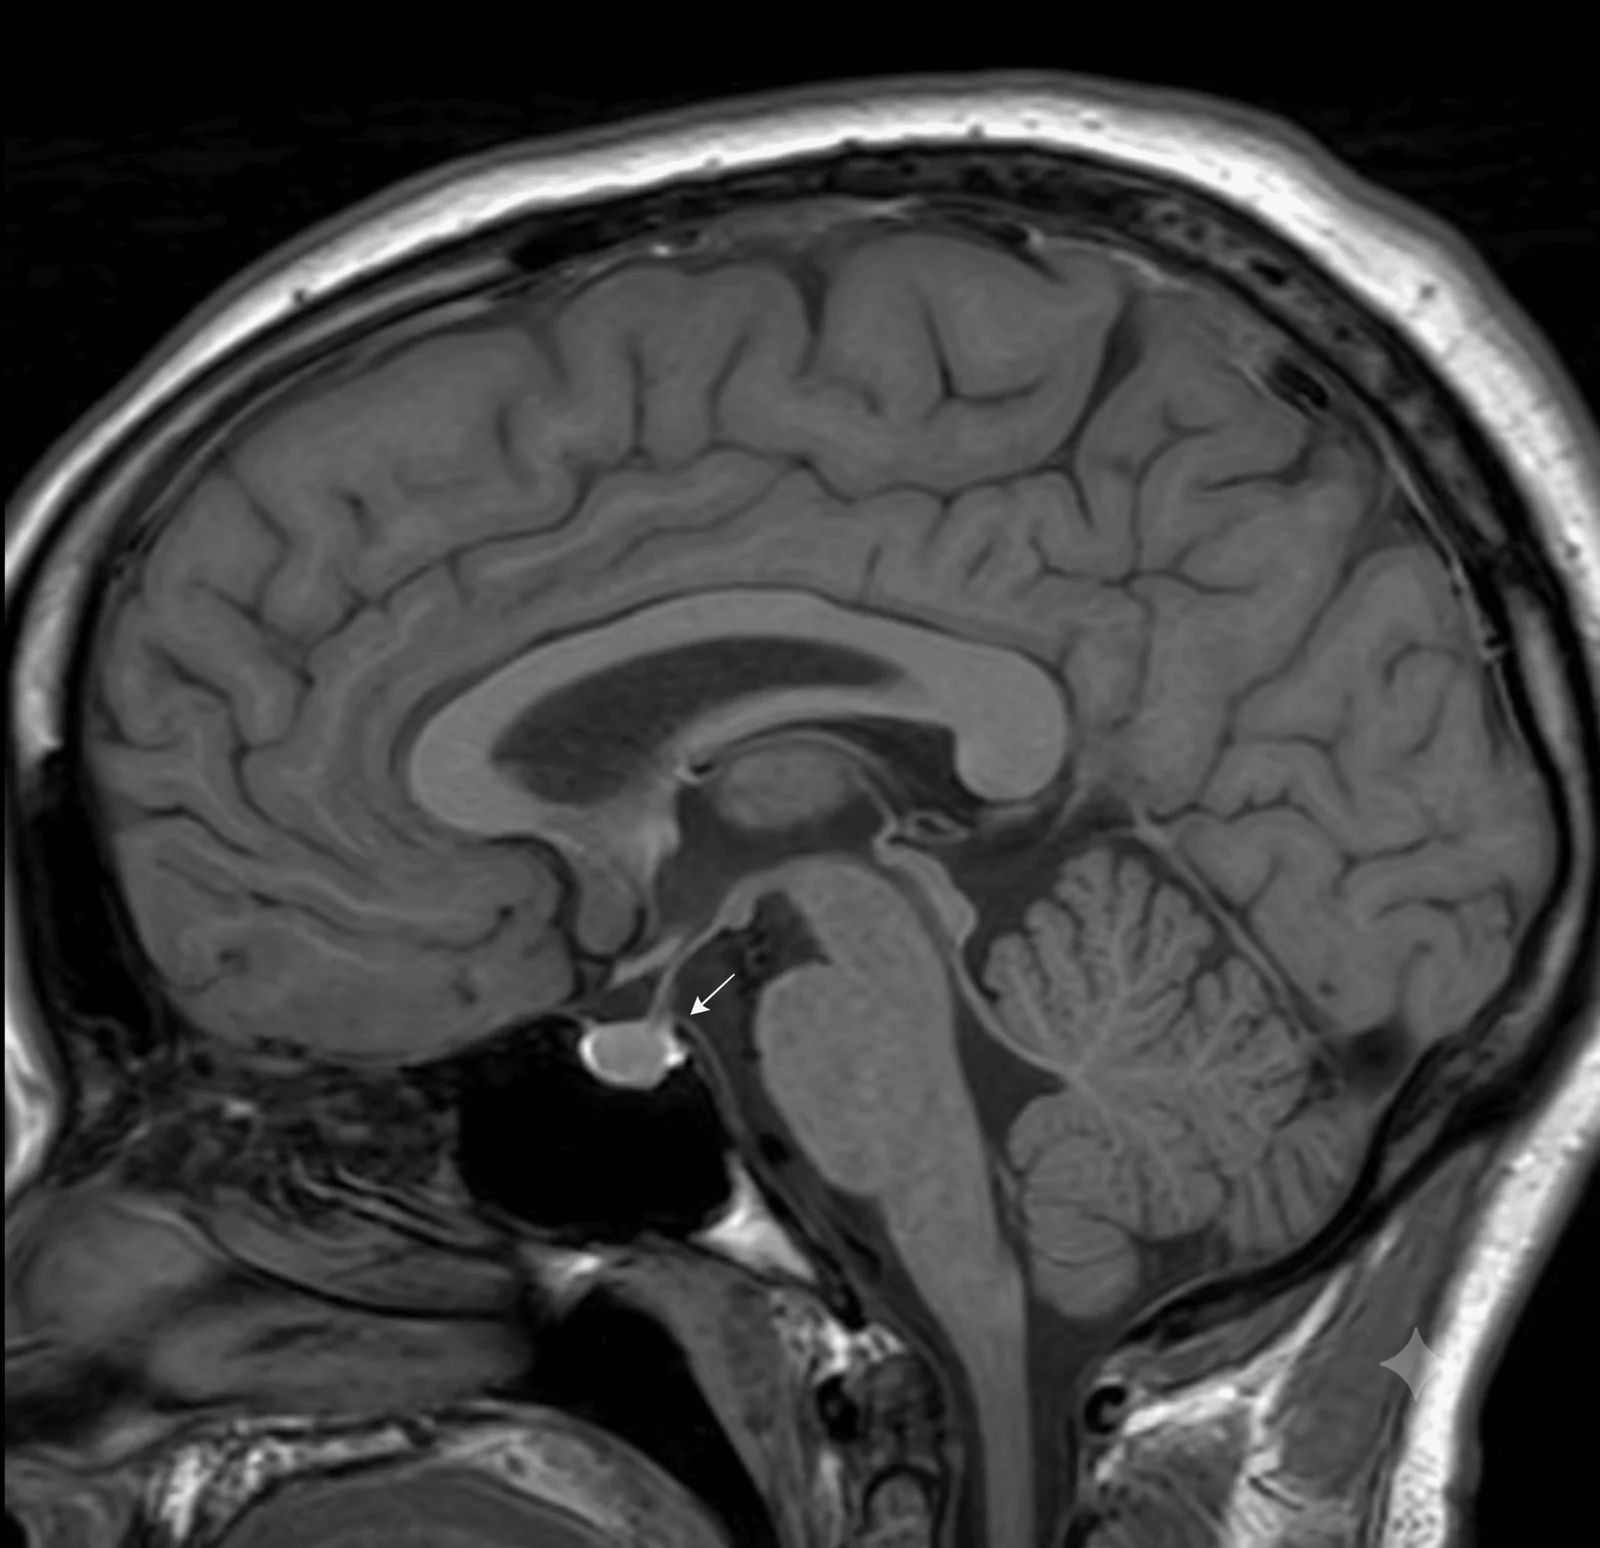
MRI of the sella turcica. Normal-sized sella turcica with an absence of identifiable adenohypophyseal tissue, confirming the diagnosis of empty sella (white arrow).
